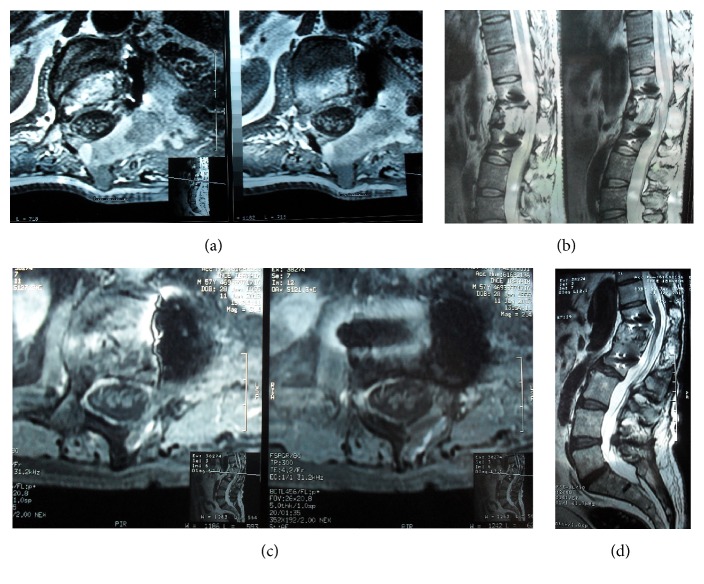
(a, b) MRI, axial T_1_WI and sagittal T_2_WI: the hemangioma is totally removed and the spinal cord is decompressed. (c, d) At the sixteenth year follow-up MRI, axial T_1_WI with gadolinium and sagittal T_2_WI show no recurrence.
